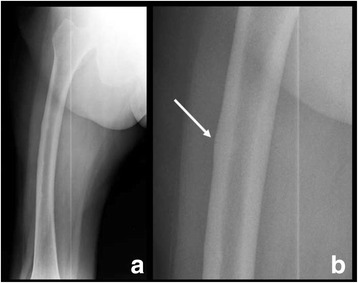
Radiograph of a patient with an incomplete AFF (a and b, detail). a. Note the femoral bowing. b. Note the location of the fracture line on the lateral cortex,and the focal cortical thickening at the fracture site
